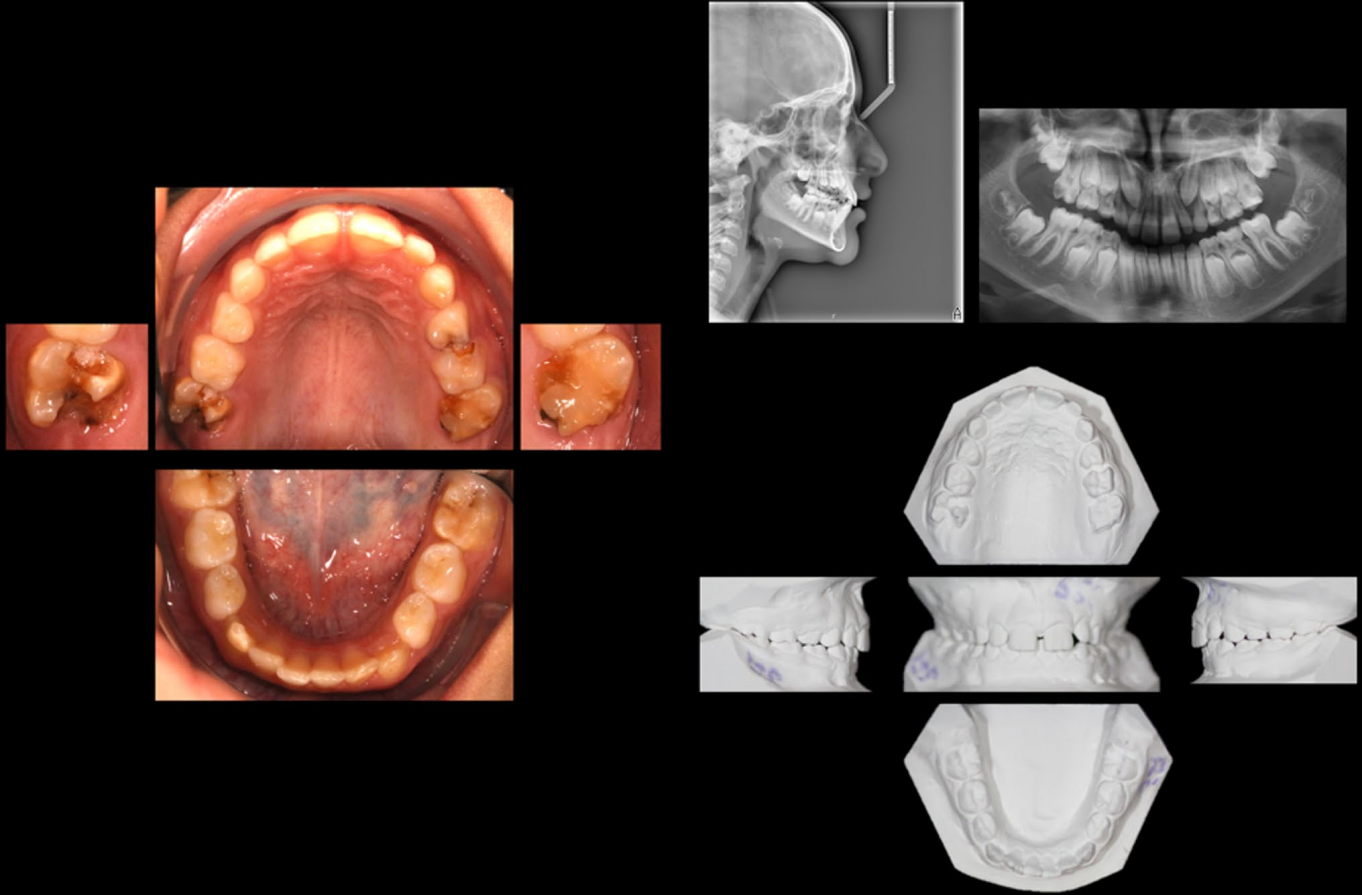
Patient case example including clinical intraoral photos, profile and panoramic radiographs, and photos of plaster models
